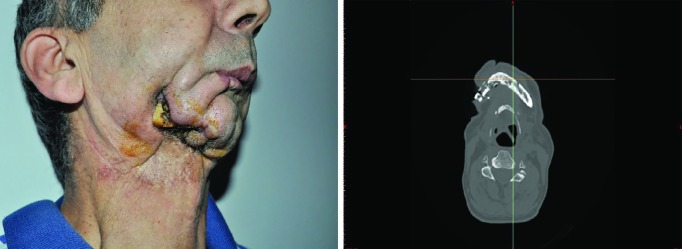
Clinical photo and CT scan of a patient with osteoradionecrosis
